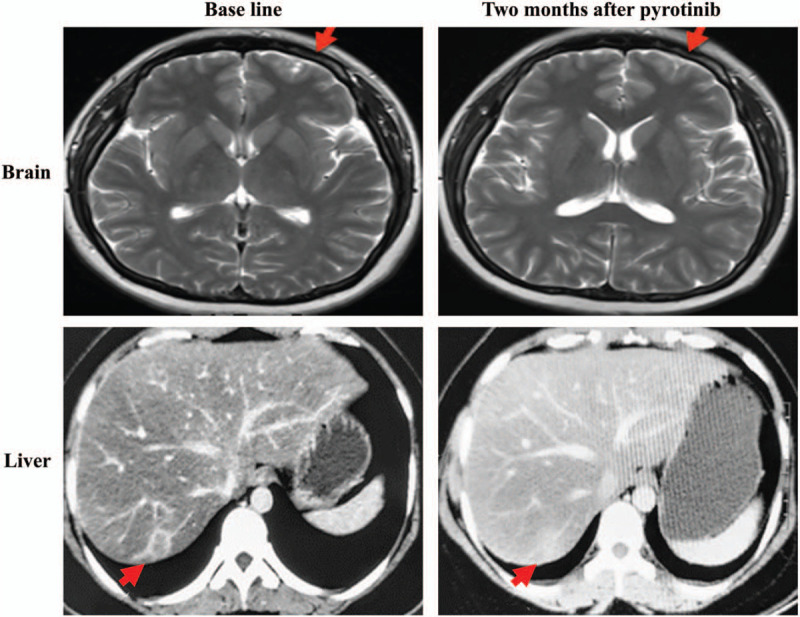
The magnetic resonance imaging of the brain and the computed tomography images (CT) of the liver before and after pyrotinib treatment. Red arrows represent the tumors.
